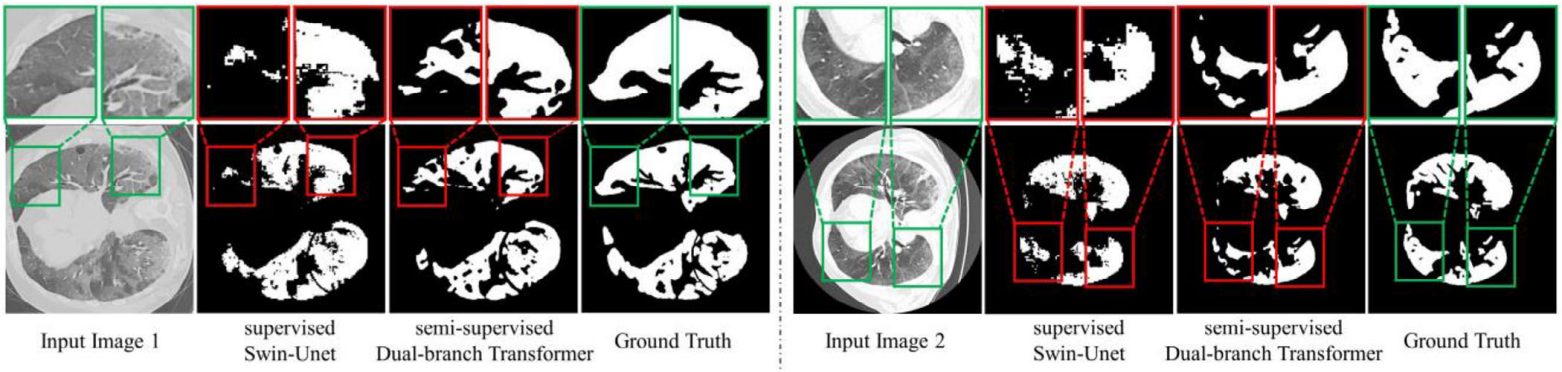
Examples of the segmentation performance of the proposed semi‐supervised Dual‐branch Transformer and the fully supervised model Swin‐Unet on the COVID‐19 CT dataset. For semi‐supervised learning, 20% of the training set are labeled, and the remaining 80% are unlabeled. The first row shows an enlarged view of the boxed region in the second row. It can be seen that our semi‐supervised method provides a more precise characterization of shape features due to the incorporation of geometric constraints.
